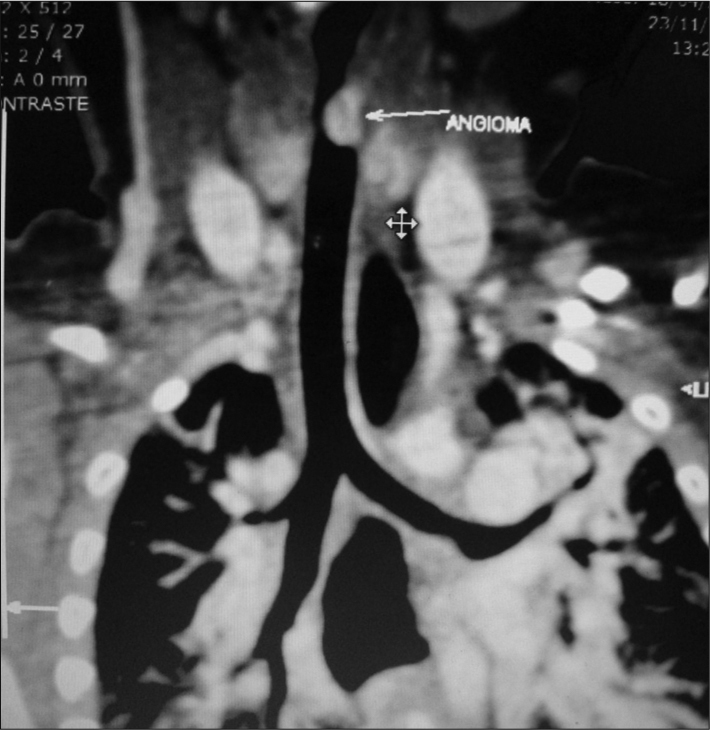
Chest and neck CT scan showing an angioma
